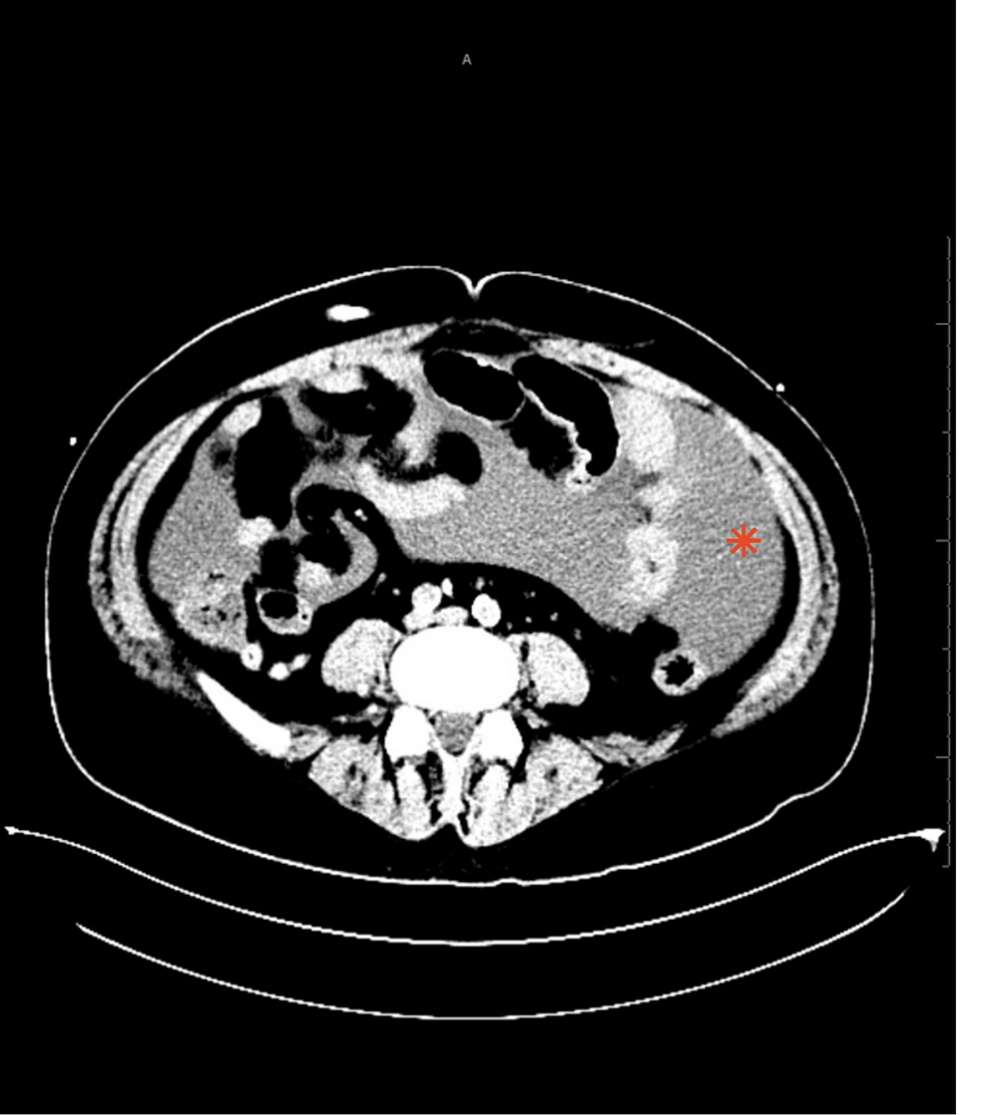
CT abdomen axial view showing the peritoneal fluid collection - Indicated by asterisk
